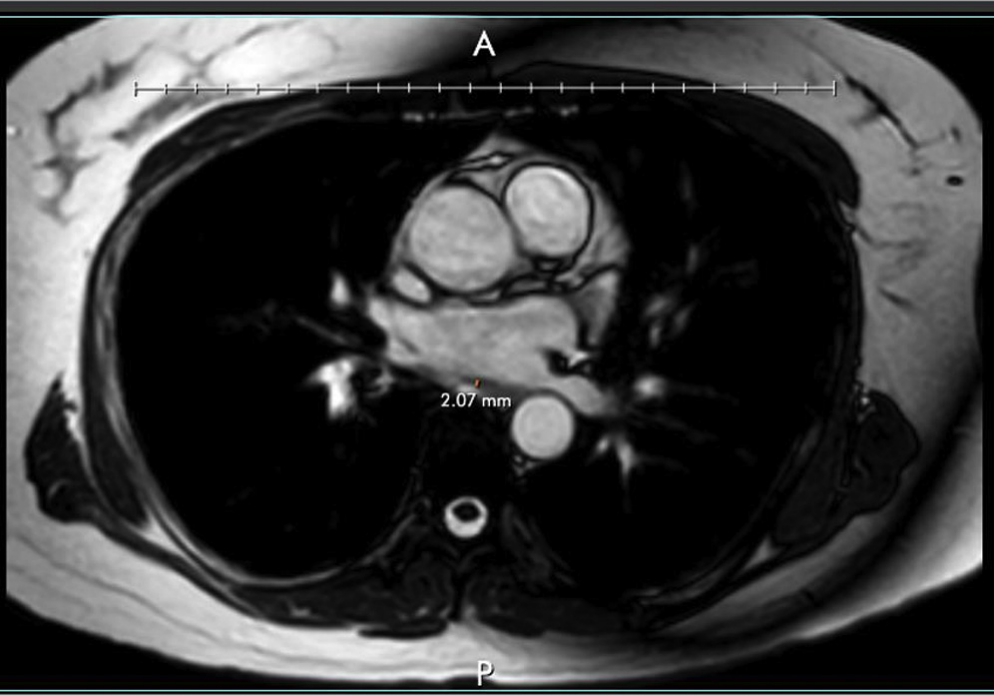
Example of left atrial–esophageal distance measurement based on cardiac magnetic resonance imaging.
